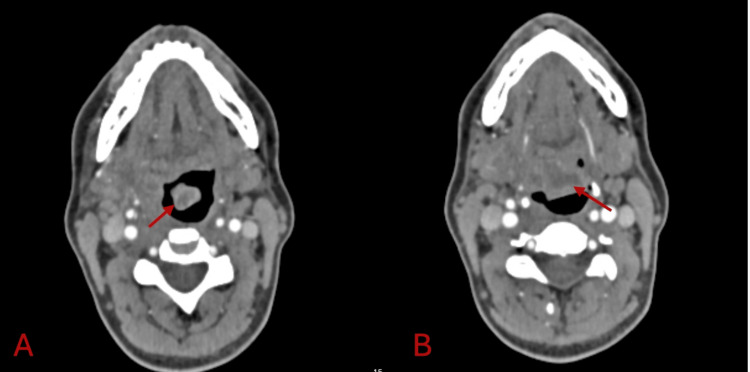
Axial contrast enhanced CT scan images of the neck. (A) Superior aspect of epiglottic abscess with arrow indicating the right aspect. (B) Inferior extent of the epiglottic abscess, extending to the right tongue base, with arrow indicating the main bulk of the abscess.
